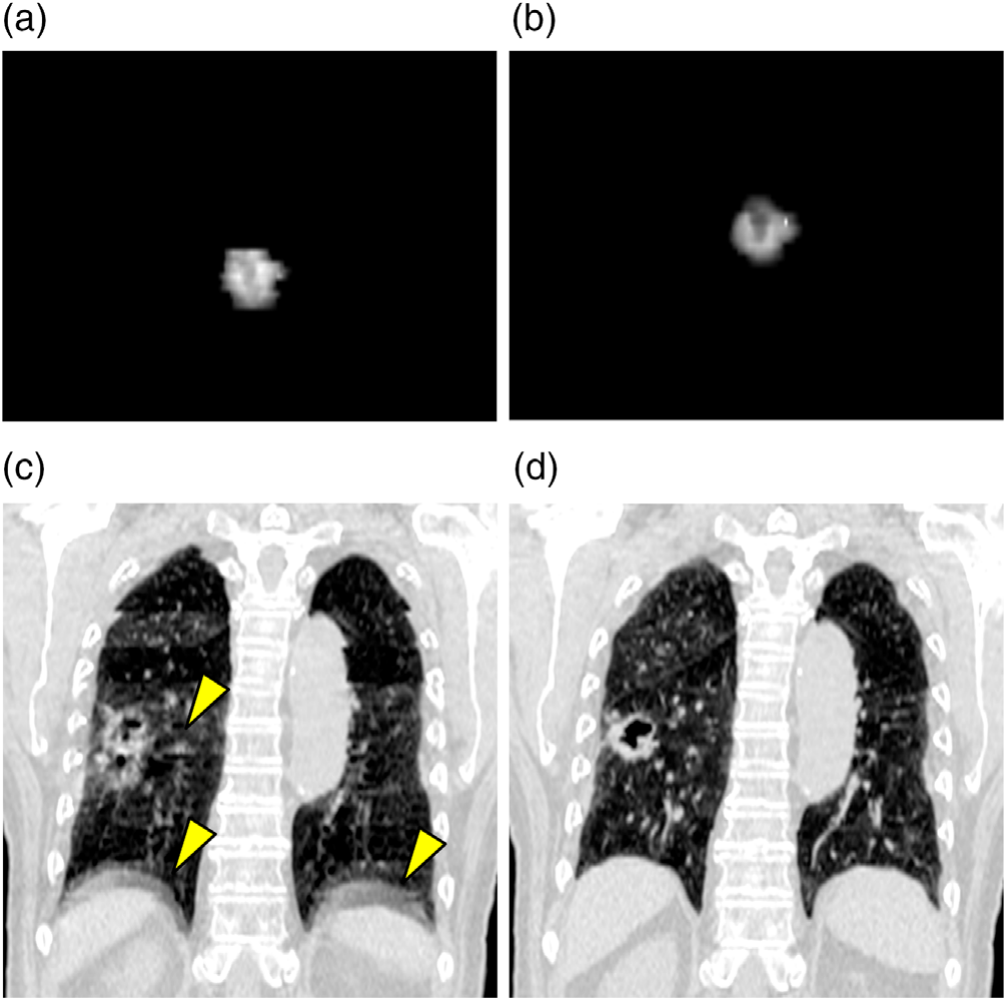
Demonstration of artifacts and inconsistent GTV contours on DRR images and CT volume of Patient 2. (a) DRR at end of inhalation, X‐ray tube angle at 275°; (b) DRR at end of exhalation; X‐ray tube angle at 275°; (c) Coronal view of CT at end of inhalation, yellow arrows indicate artifacts; (d) Coronal view of CT at end of exhalation
